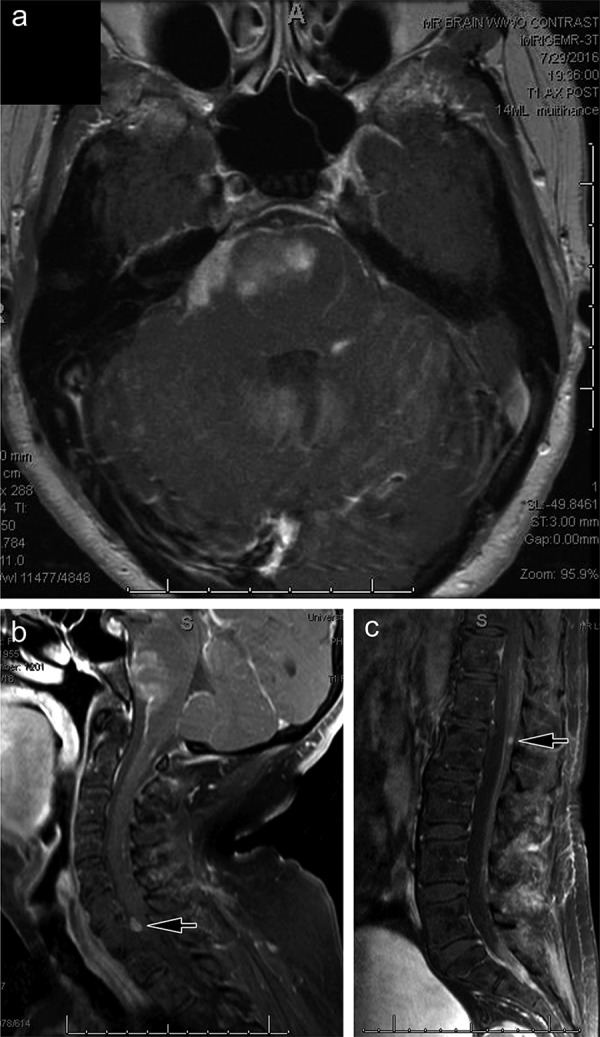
Adult diffuse midline gliomas can present with metastatic lesions. a: Contrast-enhanced axial T1-weighted MR image demonstrates a diffuse infiltrating lesion of the pons in a 61-year-old female patient (patient 10). b, c: Contrast-enhanced sagittal T1-weighted MR image of the spinal cord demonstrating leptomeningeal metastases at diagnosis (arrows).
